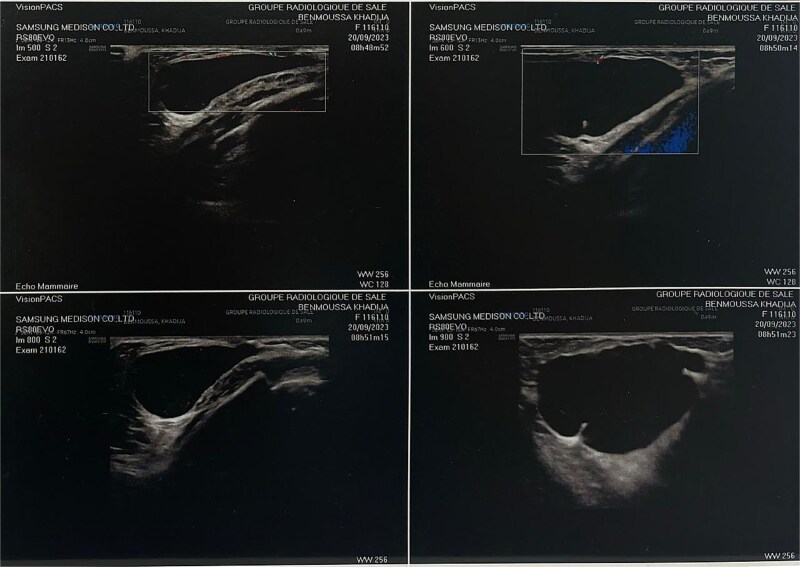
Ultrasonography of left breast showing a cystic lesion.
